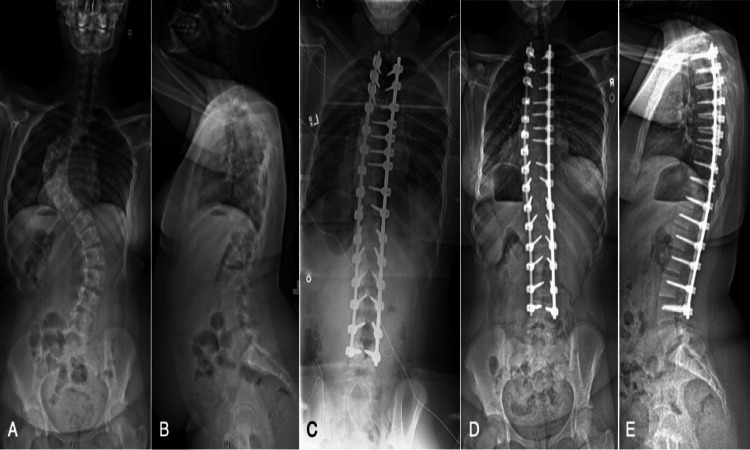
The pre- and post-operative radiographs of Case 1. Preoperative standing posteroanterior (A) and lateral (B) radiographs of a 13-year-old female with a maximum coronal Cobb of 90° with apex at T11 and a sagittal Cobb of 30° with apex at T7. Intraoperative posteroanterior radiograph (C) demonstrating reduction of the coronal deformity with a pedicle screw construct from T2-L3. Eight-month postoperative standing posteroanterior (D) and lateral (E) radiographs.
